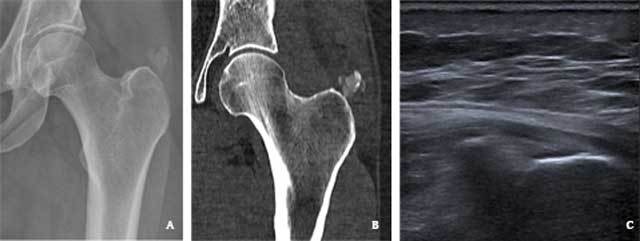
A 46-year-old woman with calcific tendinitis of the left gluteus medius tendon. (A) Plain radiography reveals a large calcification superior to the greater trochanter (B) CT with soft tissue window confirms this calcification (arrow), and locates it in the gluteus medius tendon. (C) Ultrasound image confirms a hyperechogenic calcification in the gluteus medius tendon.
